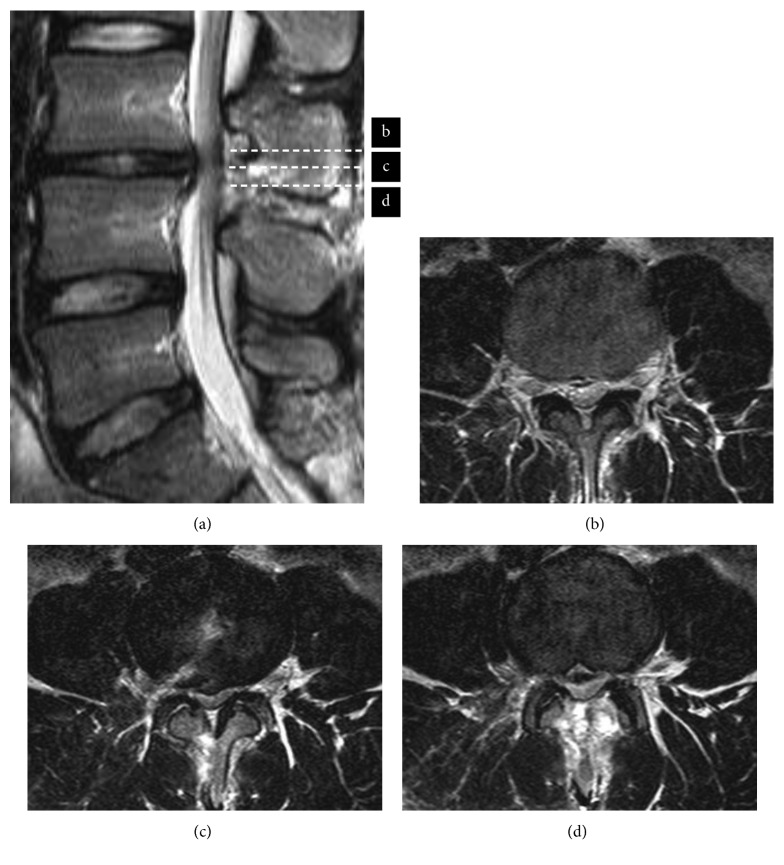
The latest postoperative T2-weighted MRI showed the removal of the nucleus pulposus and distension of the dura mater (a–d).
